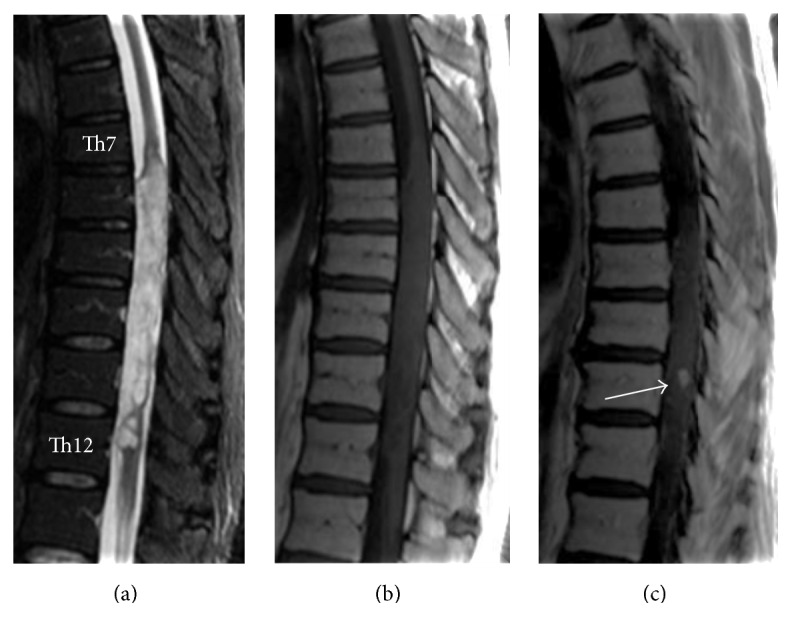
(a) Sagittal T2W1 showing cord expansion and hyperintense signal extending from the Th7 level to Th12 level surrounding both anterior and posterior aspects of cord. (b) Sagittal T1W1 showing an isointense mass with no clear-cut demarcation between cord and tumor. (c) Gadolinium contrast-enhanced MRI reveals slight enhancement. This is only a part of the tumor.
